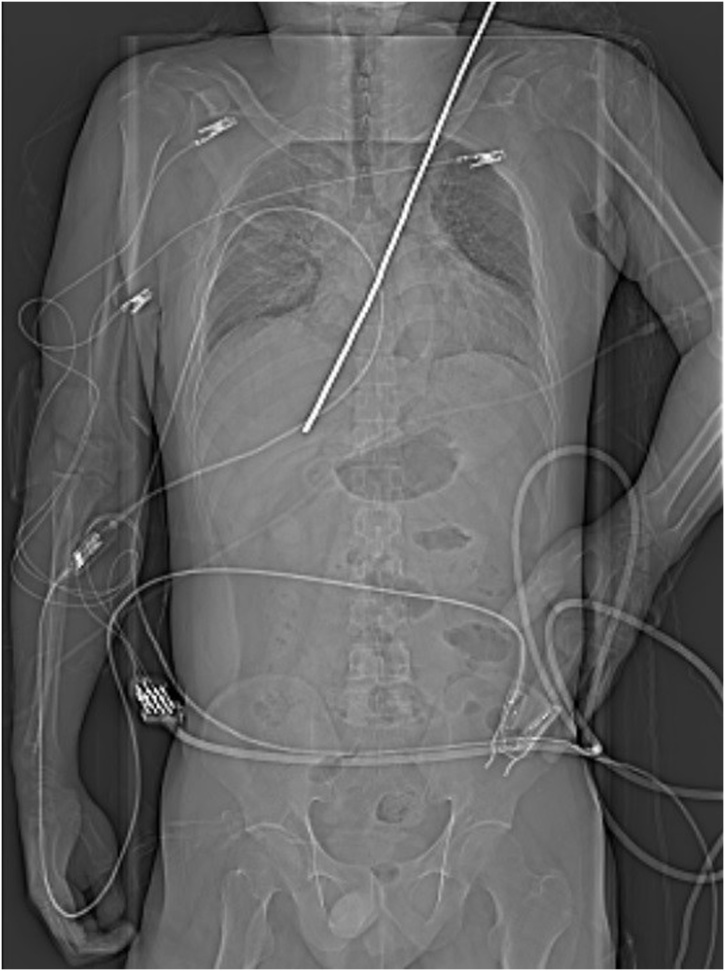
Chest computed tomography scan showing the steel rebar penetrating through the left shoulder into the right atrium.
